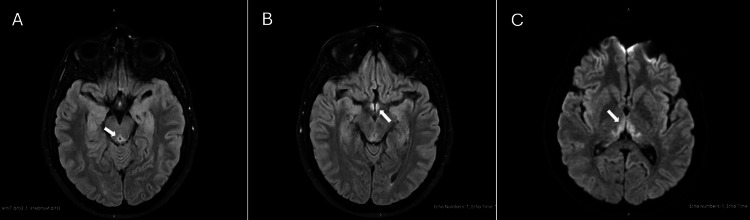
Magnetic resonance images of the patient’s brain. MRI brain showing high-signal intensity alterations in the periaqueductal gray (A, white arrow), the mamillary body (B, white arrow), and the bilateral thalami (C, white arrow).
